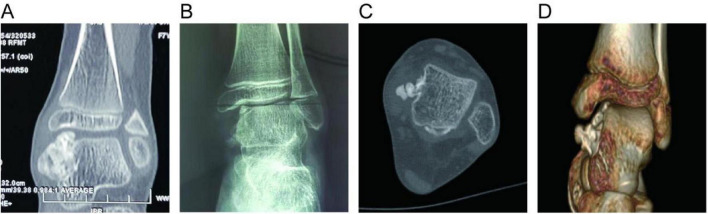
(A) Initially, a CT plain scan reveals an irregular mass of diffusely proliferating tissue in the left talus. The texture is uneven, and the posteromedial bony cortex of the talus appears blurred, exhibiting signs of destruction. (B) Following the first operation, a digital radiograph (DR) shows a cloud-like shadow at the medial margin of the talus. (C,D) On the recurrent CT plain scan and three-dimensional reconstruction, an irregularly shaped elevation with inconsistent bone density is observed at the medial margin of the talus, presenting a lobulated appearance. Its volume is smaller compared to the initial onset.
